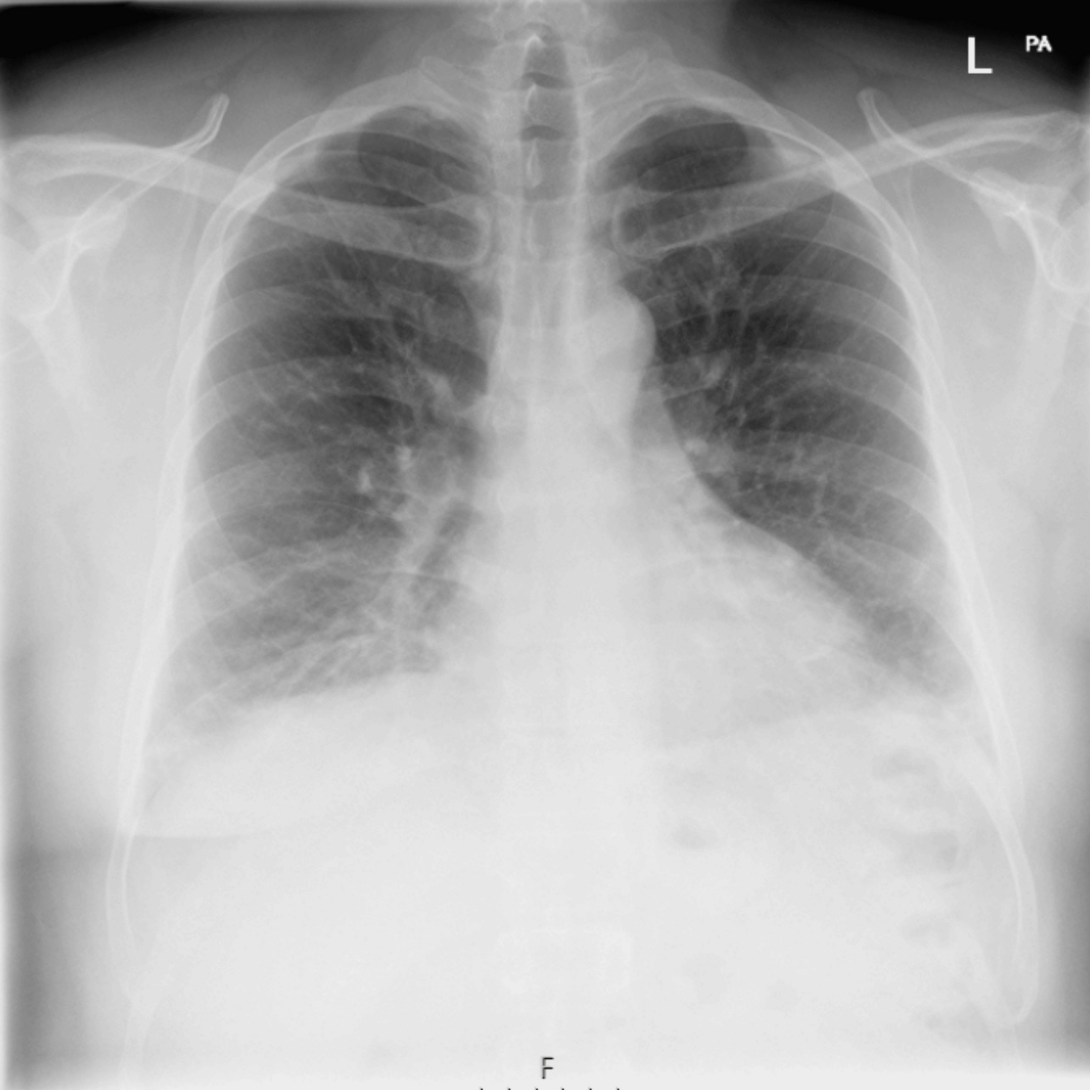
Chest X-ray: Bilateral basal consolidations, and reticular changes.
